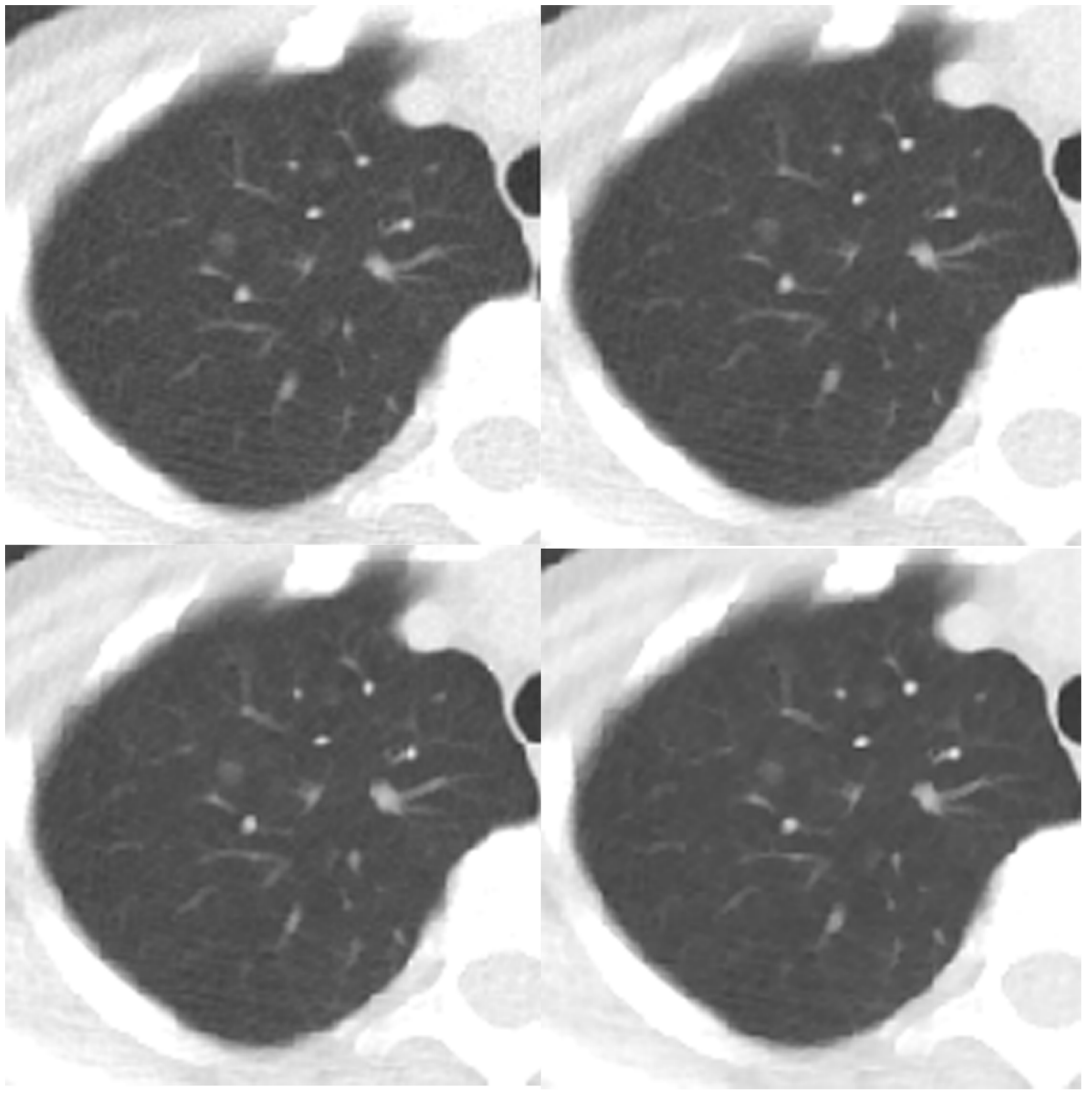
Transverse chest CT images of a patient who had a ground glass opacity nodule in the right upper lobe (arrow). Images are all NI = 30 and (A) 0% ASIR, objective image noise 32.37 HU; (B) 30% ASIR, objective image noise 25.90 HU, (C) 50% ASIR, objective image noise 22.15 HU, and (D) 80% ASIR, objective image noise 16.66 HU. The visibility of the small structure in D was higher than in A, and the margin of the lesion in D was clearer than in A.
